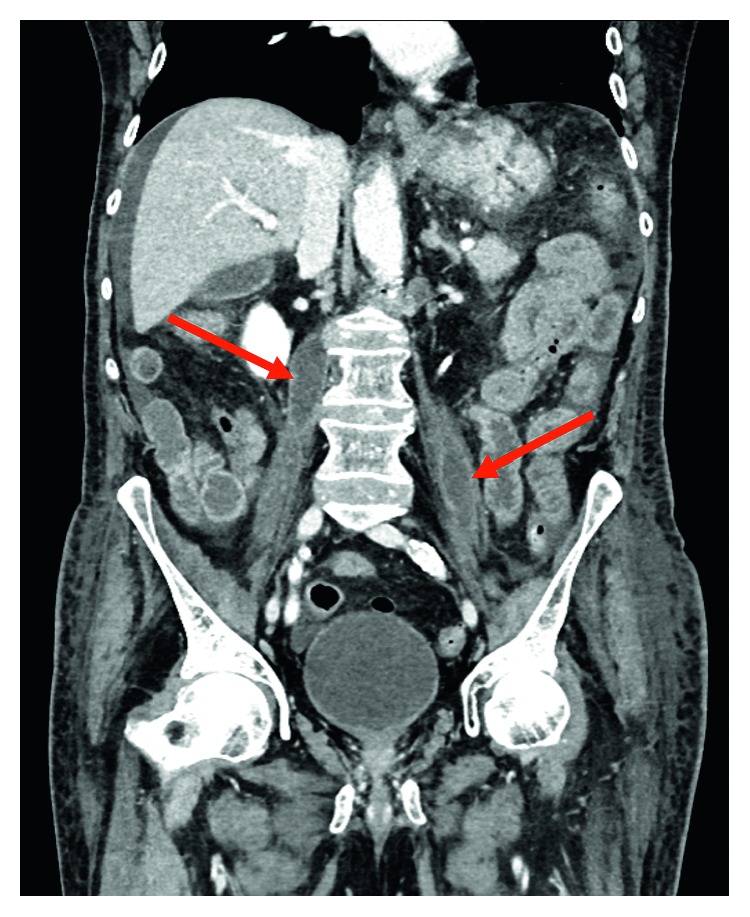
Coronal IV contrast-enhanced CT demonstrating bilateral psoas abscesses (red arrows).
